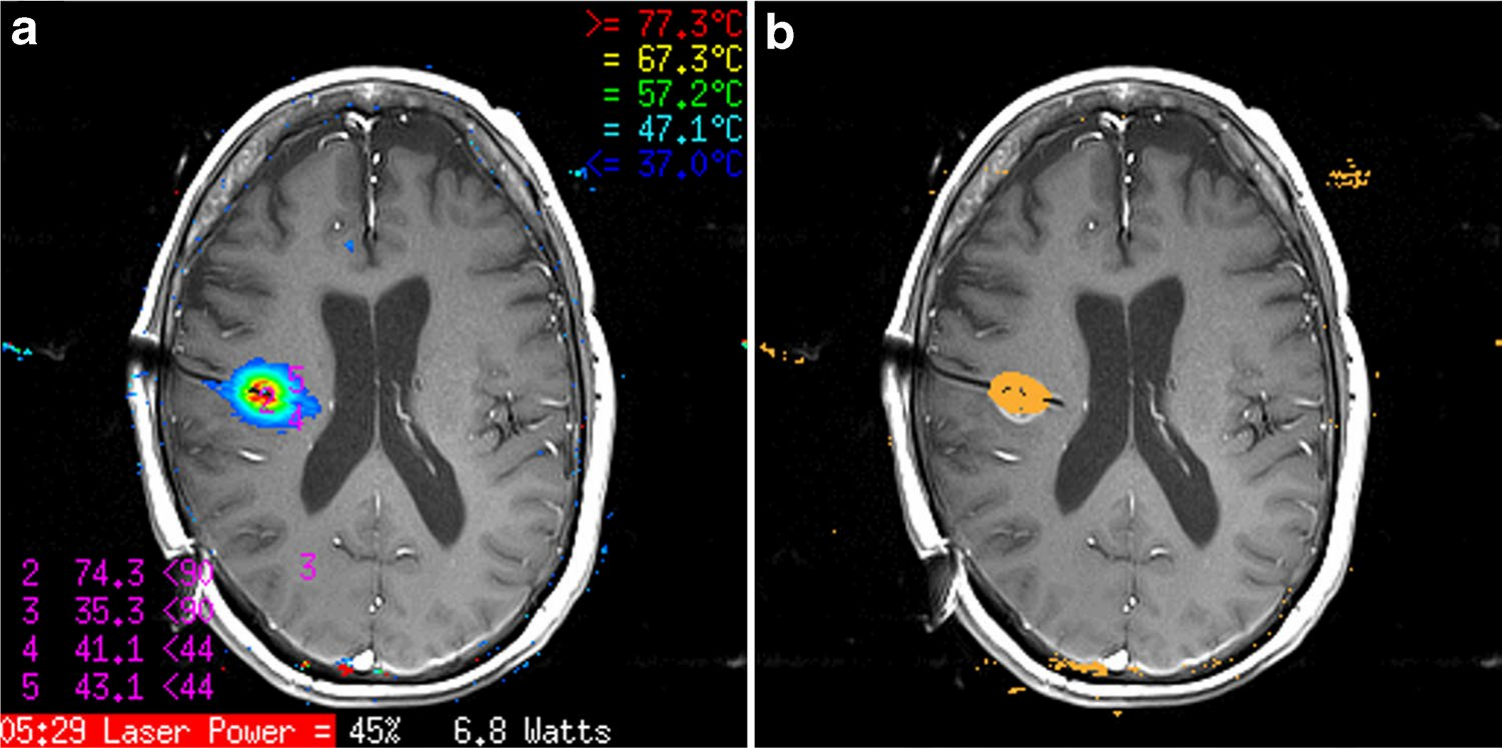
Images of tumor ablation after stereotactic frame-based and drill guide-aided brain biopsy and laser probe insertion. a Axial contrast-enhanced real-time magnetic resonance imaging for thermometry. b Monitoring of thermal damage during the magnetic resonance imaging-guided interstitial thermal therapy procedure with a 10-mm fiber core laser applicator
